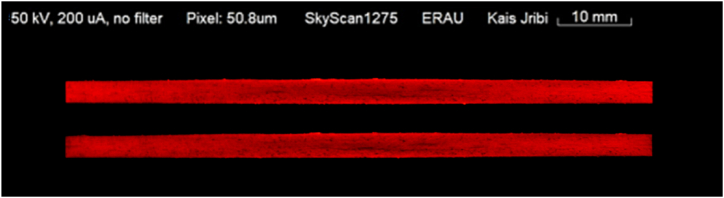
CT-Scan of a sample before and after CAI with 0.75 mm induced displacement (top: pre-CAI, bottom: post-CAI)), showing the increase in the delamination areas.
